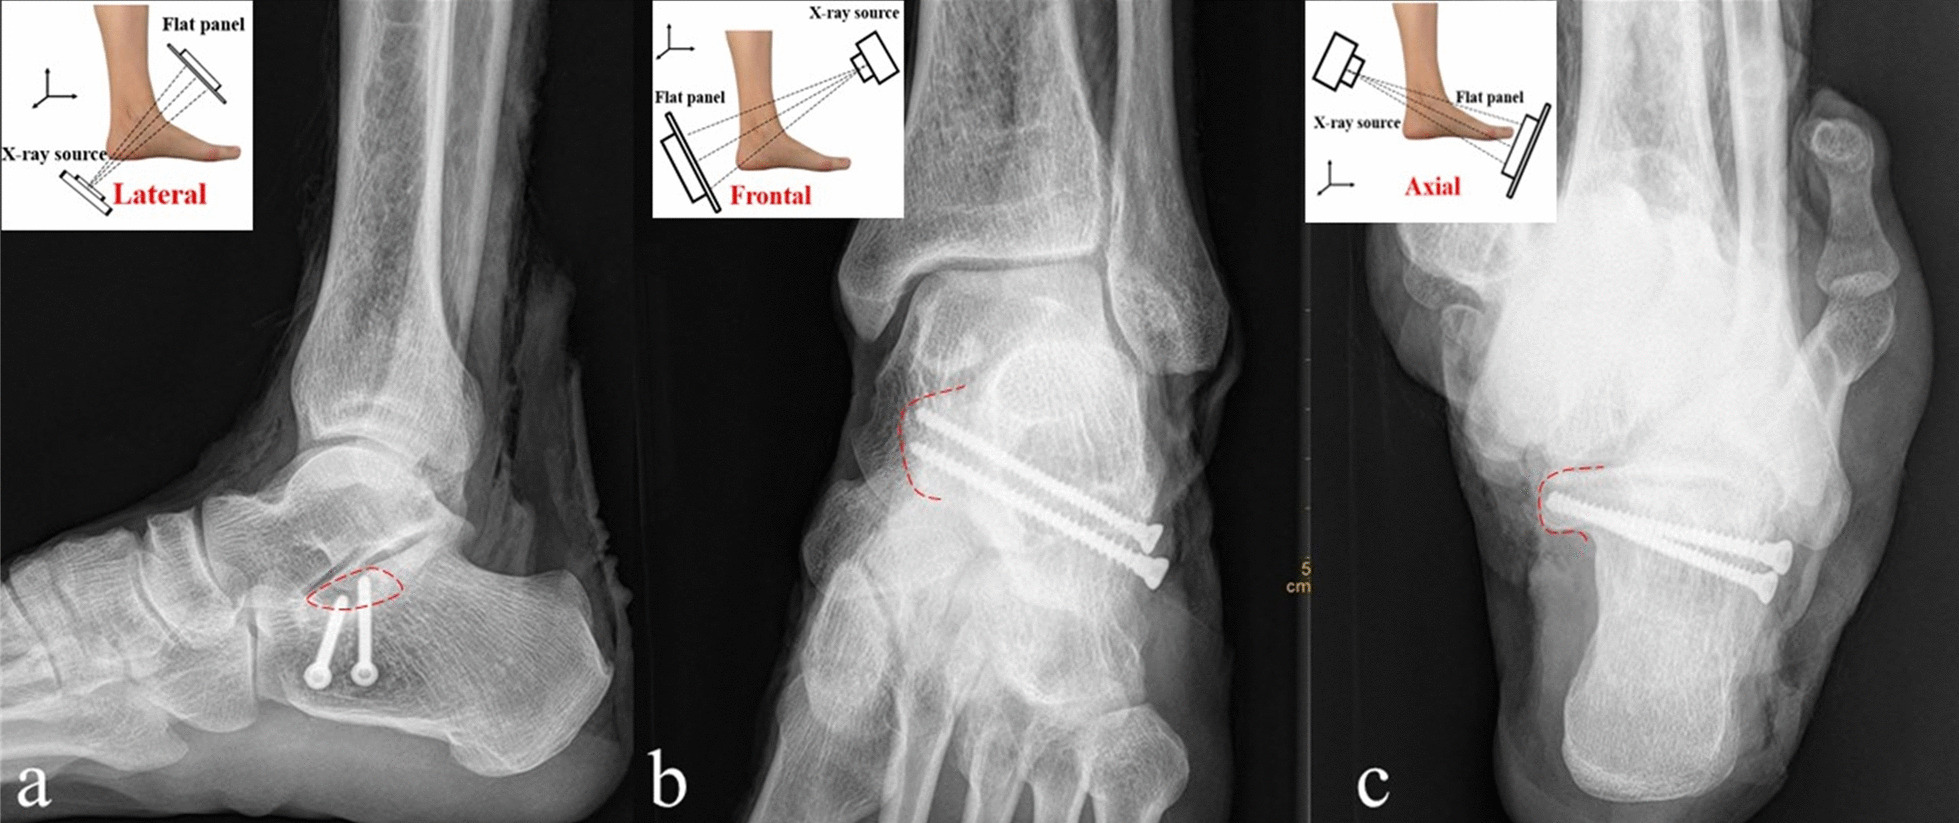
Sustentacular screw X-ray image. The red dotted line marks the outline of sustentaculum tali. a: Lateral X-ray of the ankle; b: frontal X-ray image of the sustentaculum tali; c: axial X-ray image of the sustentaculum tali
